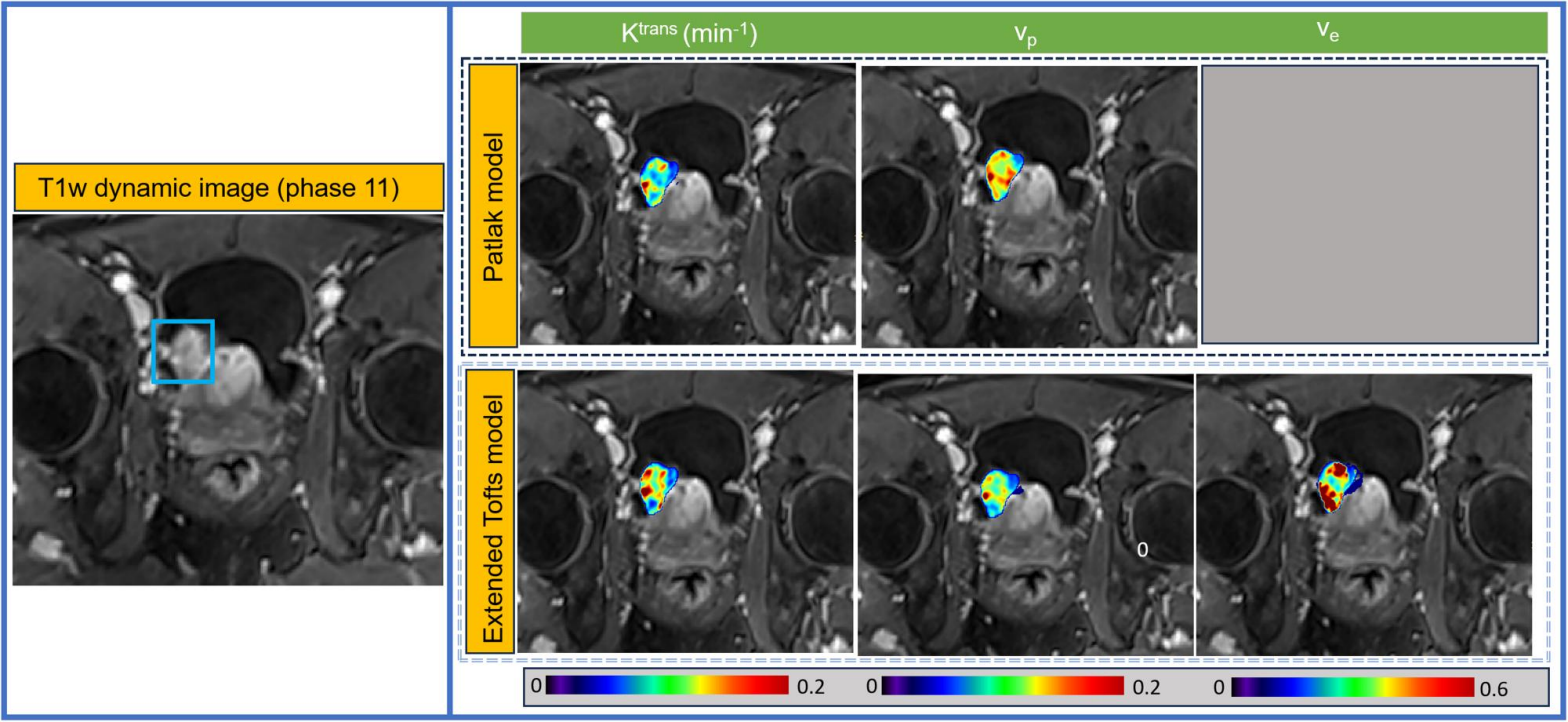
MRI-MBA (Model-Based Analysis) includes algorithms to analyse DCE-MRI (dynamic contrast-enhanced) data using Patlak and extended Toft models. These models derived representative parametric maps are overlaid on the post-contrast T1-weighted images.
